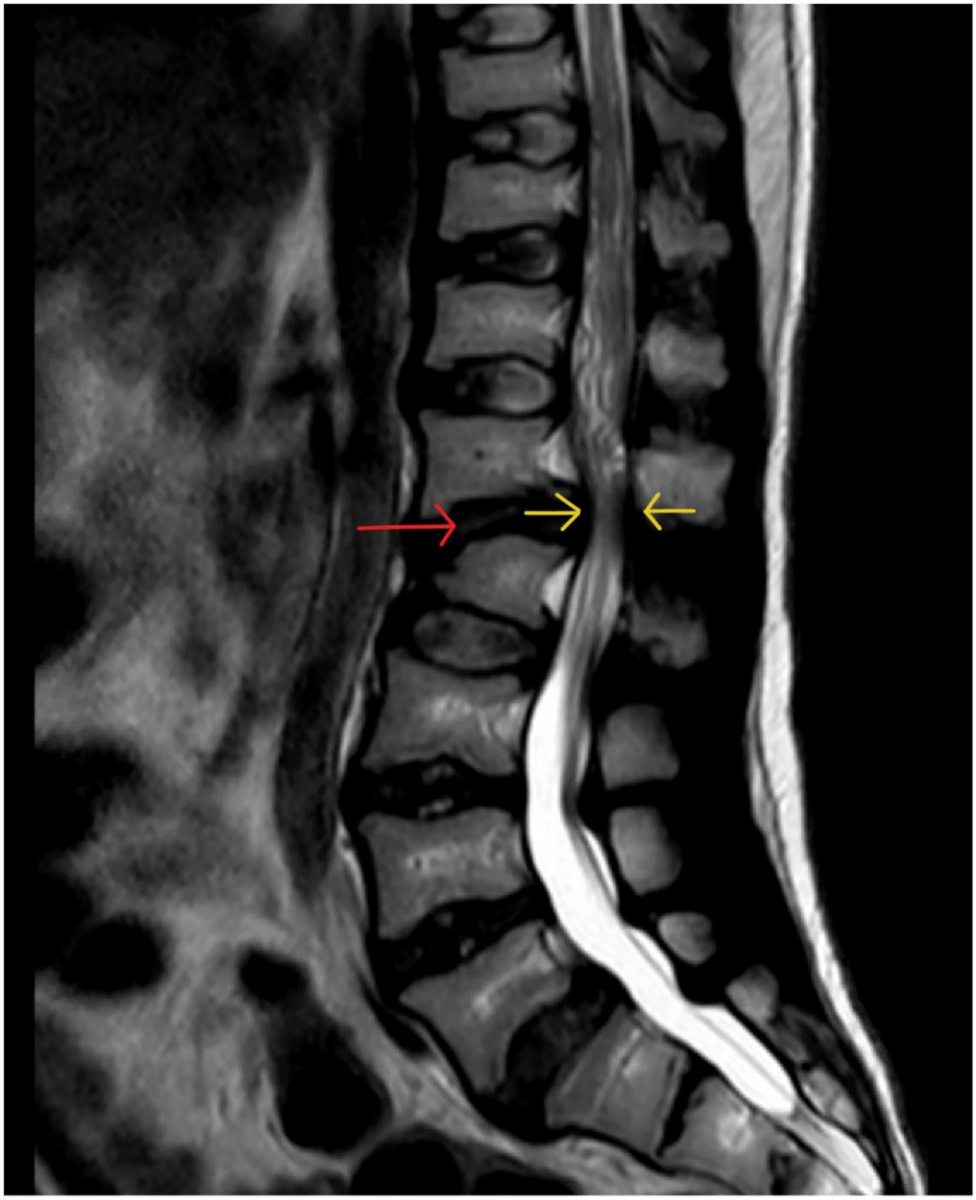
Thoraco-lumbar kyphosis. T2-weighted sagittal MRI of the adult spine with MPS VI, demonstrating abnormal vertebra body shape at L2, resulting in kyphosis at L1-L2 (red arrow) and early spinal canal compromise (yellow arrows).
